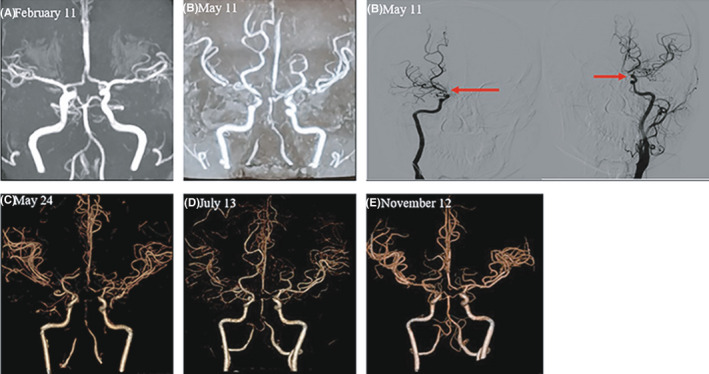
Cerebral vascular imaging before and after this onset. (A) MRA before this onset was normal. (B) MRA on the 10th day of the onset without effective antibiotic therapy indicated the bilateral varying vascular stenosis and occlusion at the beginning of internal carotid artery and middle cerebral artery, posterior cerebral artery, and adjacent collateral blood vessel formation; digital subtraction angiography (DSA) indicated multiple localized stenosis in the intracranial segment of bilateral internal carotid artery, bilateral middle cerebral artery M1 segment, and the initial segment of the basilar artery. (C) CTA on the 24th day of the onset indicated the same vascular stenosis to b. (D) CTA after the 2 months of effective anti‐infective treatment showed the improvement of vascular stenosis. (E) CTA after half a year of effective anti‐infective treatment showed obvious vascular improvement and collateral circulation formation
